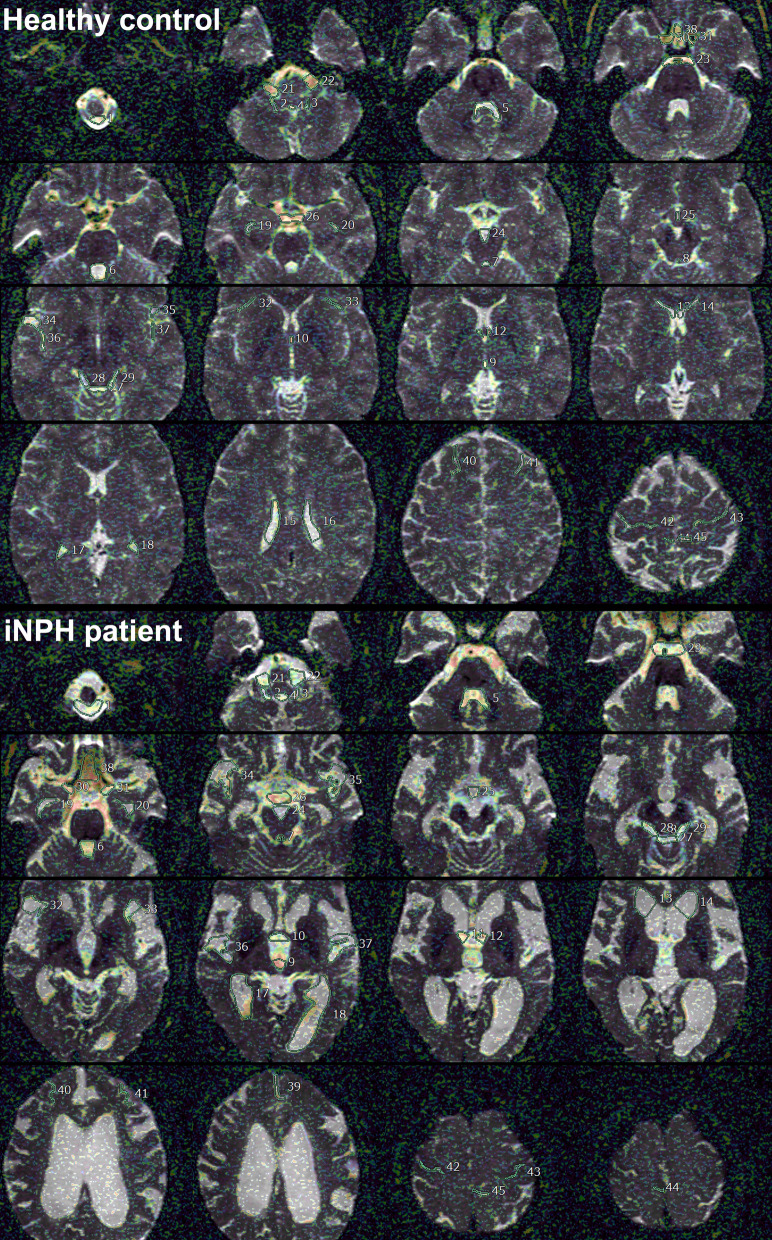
Location of the 45 regions of interest on* f* map (%) of IVIM MRI in the ventricles and subarachnoid spaces in a representative healthy control (top) and iNPH patient (bottom)
